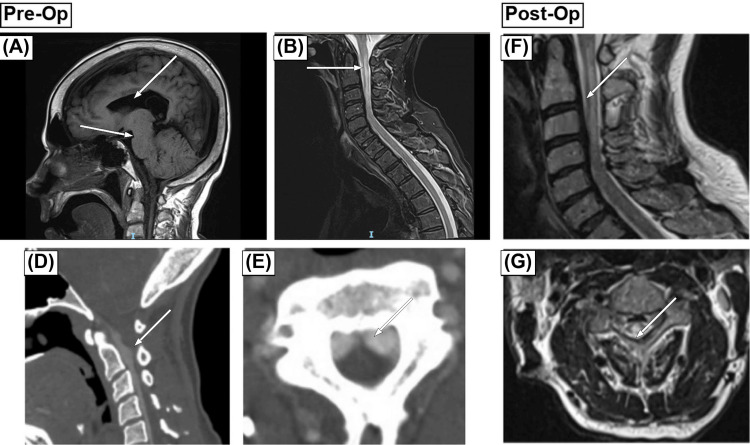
Preoperative and postoperative imaging in Case 1, a 57-year-old man with a VP shunt placed at birth for neonatal IVH. (A) Preoperative sagittal T1 brain MRI showing slit-like lateral ventricles (upper arrow) and brainstem sagging with downward displacement of the midbrain (lower arrow), consistent with chronic CSF overdrainage. (B) Preoperative sagittal T2 cervical MRI demonstrating intramedullary T2 hyperintensity at the cervico-medullary junction extending to C4 (arrow), consistent with myelopathic changes. (C) Preoperative axial T2 cervical MRI at the level of maximal compression showing intramedullary signal change consistent with myelopathy, with circumferential epidural venous plexus engorgement (arrow). (D) Preoperative sagittal CT cervical spine showing the engorged epidural venous plexus (arrow). (E) Preoperative axial CT cervical spine, late post–IV contrast, showing the engorged epidural venous plexus compressing the cord (arrow). (F) Postoperative sagittal T2 cervical MRI after shunt revision with addition of an anti-siphon device, showing resolution of the venous engorgement with persistent intramedullary T2 hyperintensity (arrow) indicating irreversible myelopathic changes due to delayed diagnosis of Miyazaki syndrome. (G) Postoperative axial T2 cervical MRI showing resolution of venous engorgement and restored perimedullary CSF spaces, with persistent intramedullary signal change (arrow) confirming irreversible myelopathy. VP, ventriculoperitoneal; IVH, neonatal intraventricular hemorrhage.
